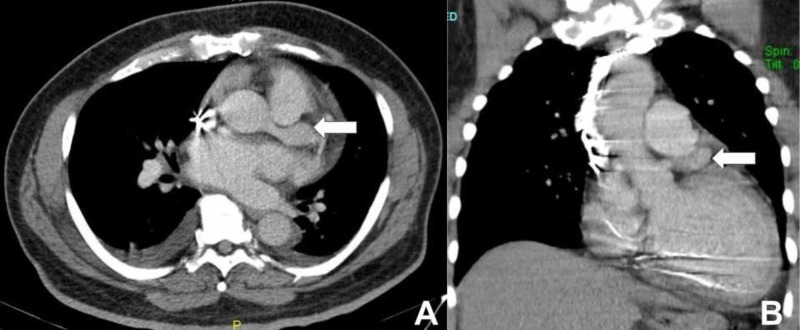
CT chest showing left main coronary artery aneurysm (white arrow) A: transverse plane; B: coronal plane
